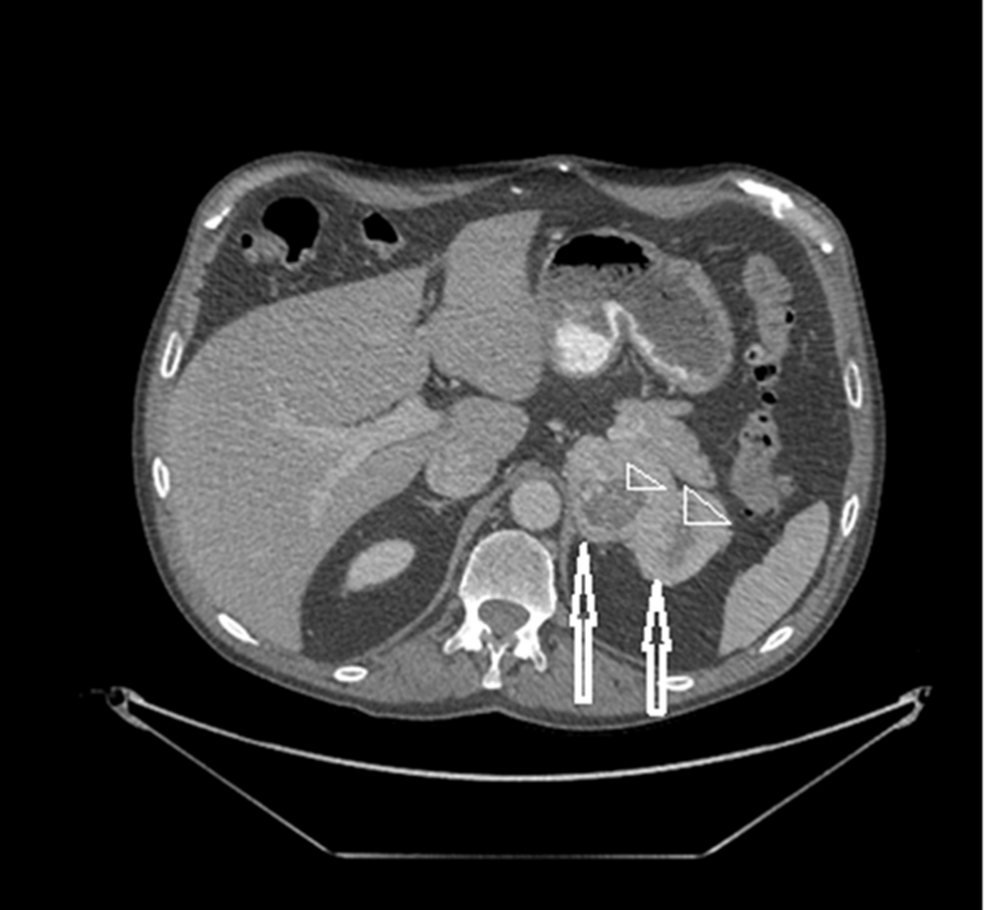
CT scan of the abdomen The image shows a bi-lobed mass (two arrows) in the left adrenal gland measuring 9 x 5.5 cm. Centrally, there are signs of low density indicating necrosis (two arrowheads) CT: computed tomography
